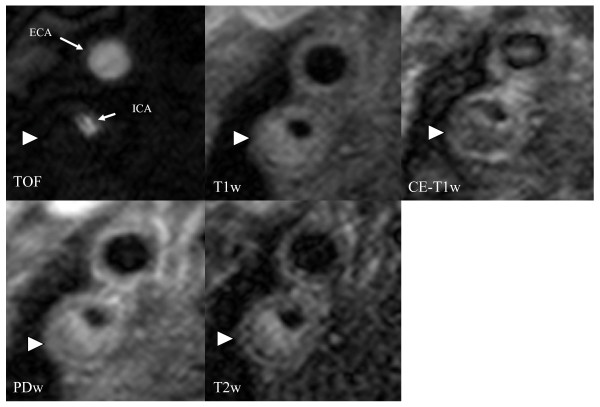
MR images of a 74 year old asymptomatic patient with a 80% carotid stenosis in his right internal carotid artery (ICA; ECA = external carotid artery). Cross- sectional MR images show a large eccentric plaque in the right ICA with a large necrotic core without intraplaque hemorrhage (arrow), which is covered by a thick layer of dense and loos fibrous tissue, which can be depicted on PD- and T2w- images as a hyperintense region near the lumen surface which shows moderate contrast enhancement on the CE-T1w images. The plaque is classified as an American Heart Association Lesion Type IV/V with a thick fibrous cap.
